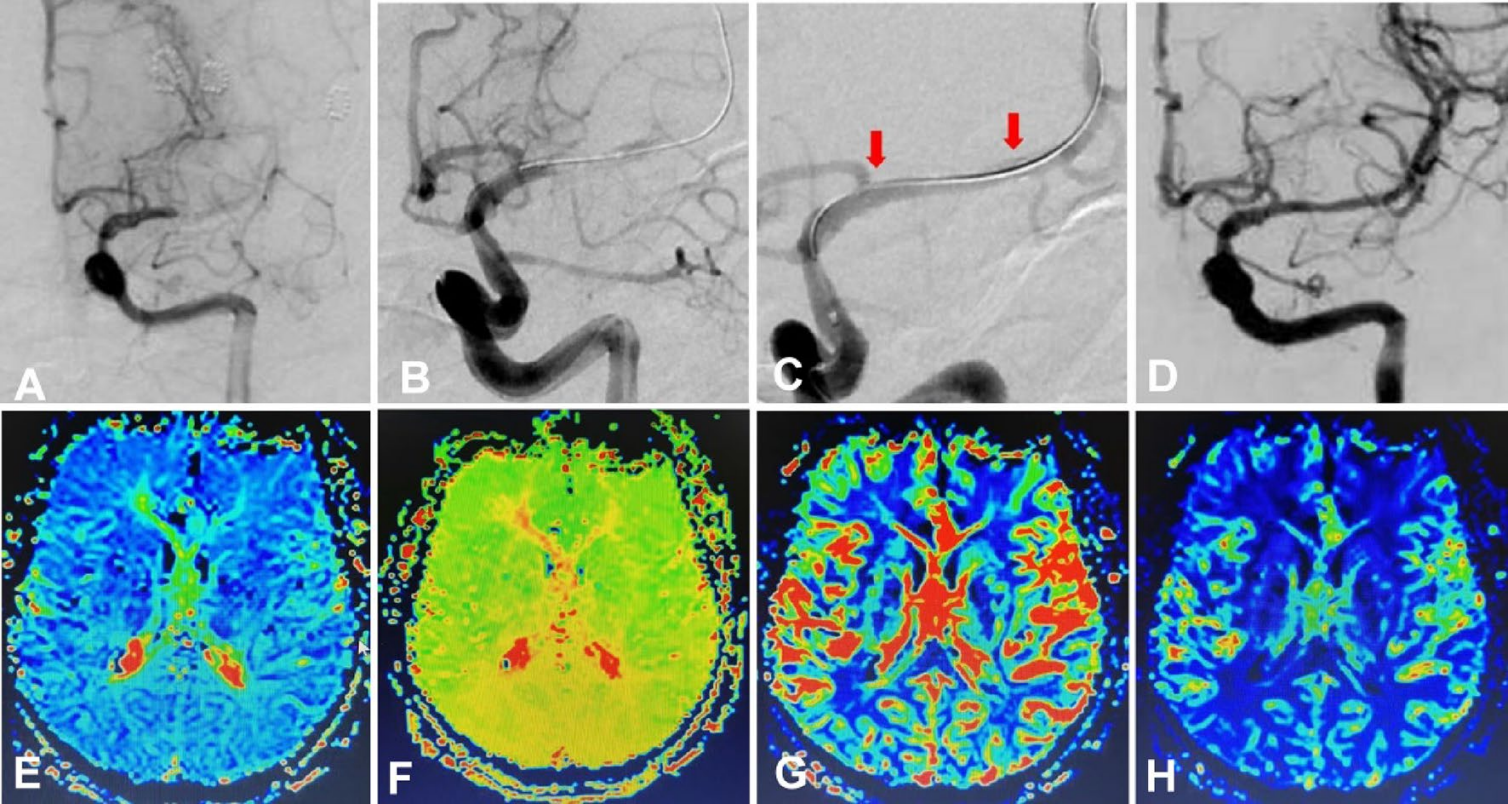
Same patient as in Fig. 2. Endovascular recanalization and magnetic resonance imaging perfusion weighted imaging (MRI PWI). (A–D) Endovascular treatment was performed 12 days after being hospitalized with a stent being deployed. (A) The M1 segment of left middle cerebral artery was occluded on digital subtraction angiography. (B) A micro-guidewire was used to explore the occluded segment. (C) An Enterprise stent (4.5 * 14 mm, Codman Neuro, New Brunswick, NJ, USA) was deployed at the occluded segment, and two red arrows indicate the markers at the proximal and distal ends of the stent. (D) At 6-month follow-up, the stented segment remained patent. (E–H) MRI PWI 5 days after operation demonstrated that bilateral perfusion was basically symmetrical and left cerebral perfusion was significantly improved. (E) Mean transit time; (F) Time to peak; (G) Cerebral blood volume; (H) cerebral blood flow. Immediately after the recanalization procedure, the modified Rankin Scale (mRS) score remained 4. At 4 month follow-up, the mRS was 1.
